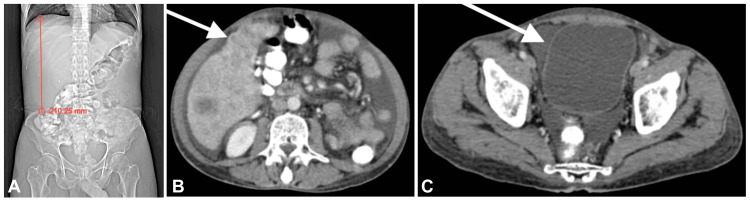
(A) A CT topogram shows an enlarged liver measuring 21 cm, indicative of hepatomegaly. (B) Contrast-enhanced CT (CECT) of the abdomen reveals nodular and irregular liver margins (white arrow), along with free fluid in the abdomen, suggestive of liver cirrhosis and ascites. (C) A CECT of the abdomen demonstrates free fluid in the pelvis (white arrow), suggestive of ascites.
